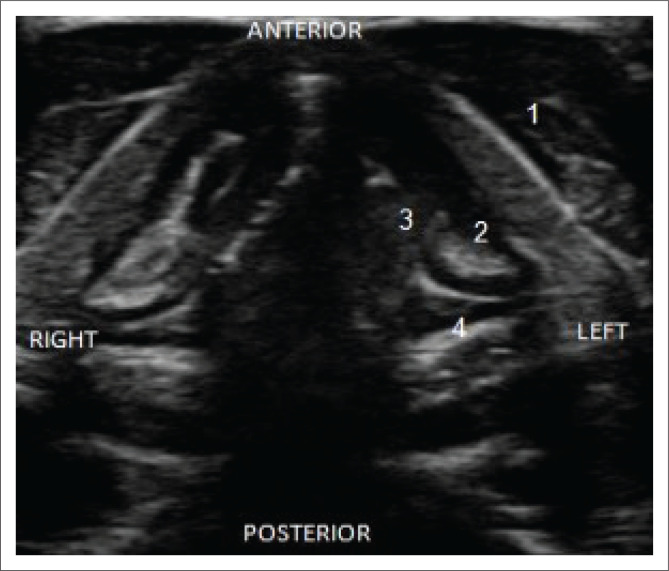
Translaryngeal ultrasonography of vocal cords in abduction where the following anatomical structures are visualised and numbered. (1) Thyroid cartilage, (2) left false vocal cord, (3) left true vocal cord and (4) arytenoid cartilage.
